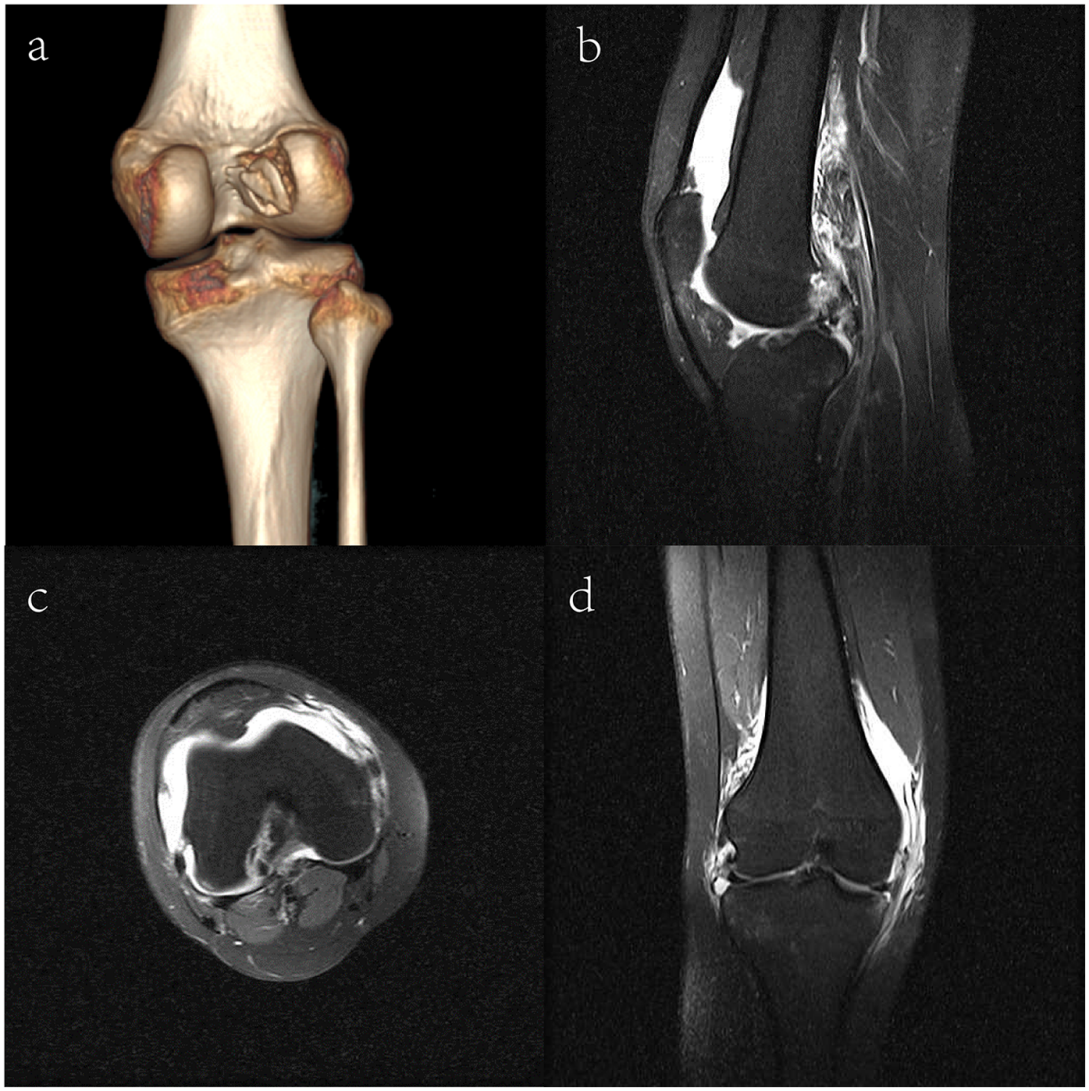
Imaging results of the patient. (a) Three-dimensional computed tomography scan; (b) Coronal view of the MRI; (c) Axial view of the MRI; (d) Sagittal view of the MRI.
